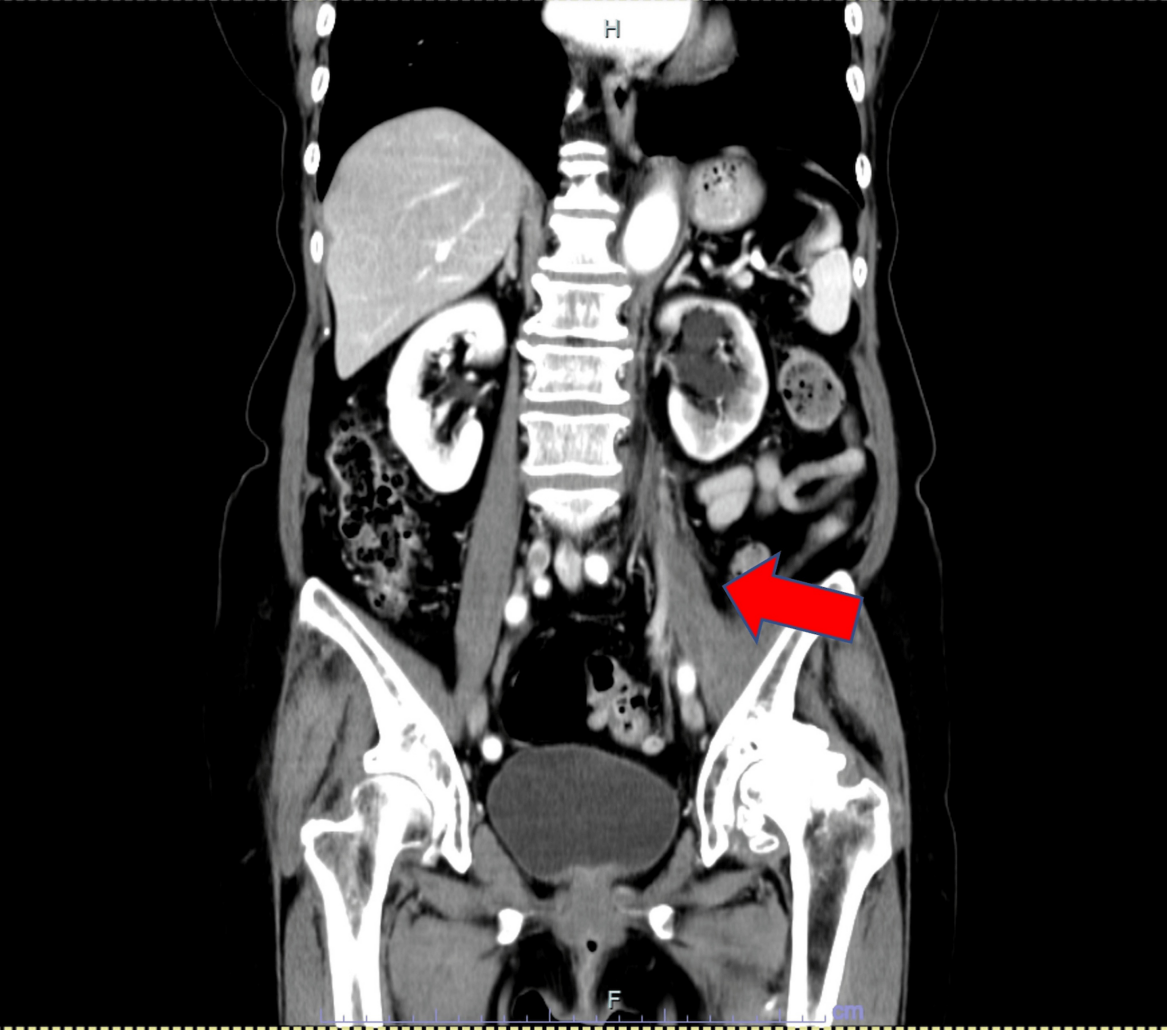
Coronal computed tomography findings. Initial treatment led to a reduction in the size of the psoas lesion to 48 × 14 mm.
